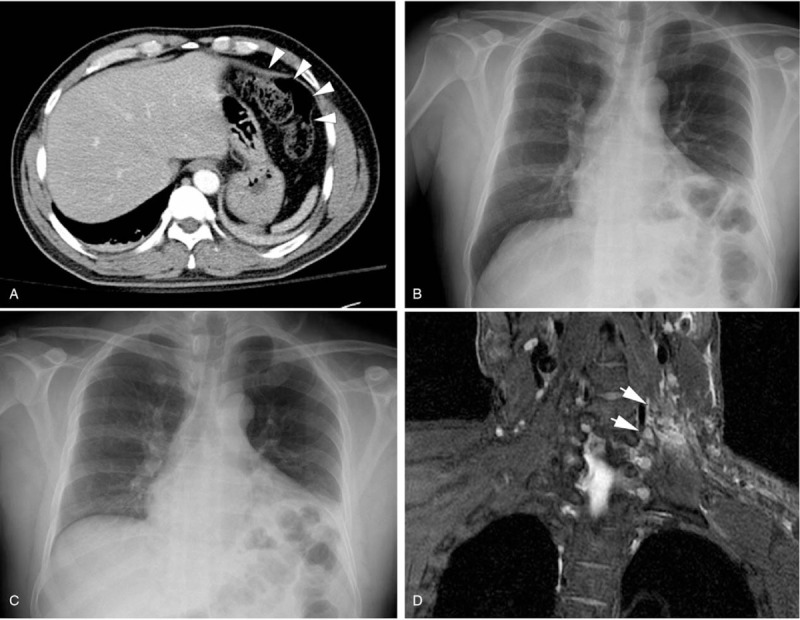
(A). Abdominal CT scan demonstrated displacement of colon to left upper quadrant (white arrowheads). (B and C). Paired inspiratory and expiratory CXRs. (D). Coronal T2-weighted MRI showed hyperintensity (white arrows) of C5-C6 nerve roots. MRI = magnetic resonance imaging.
